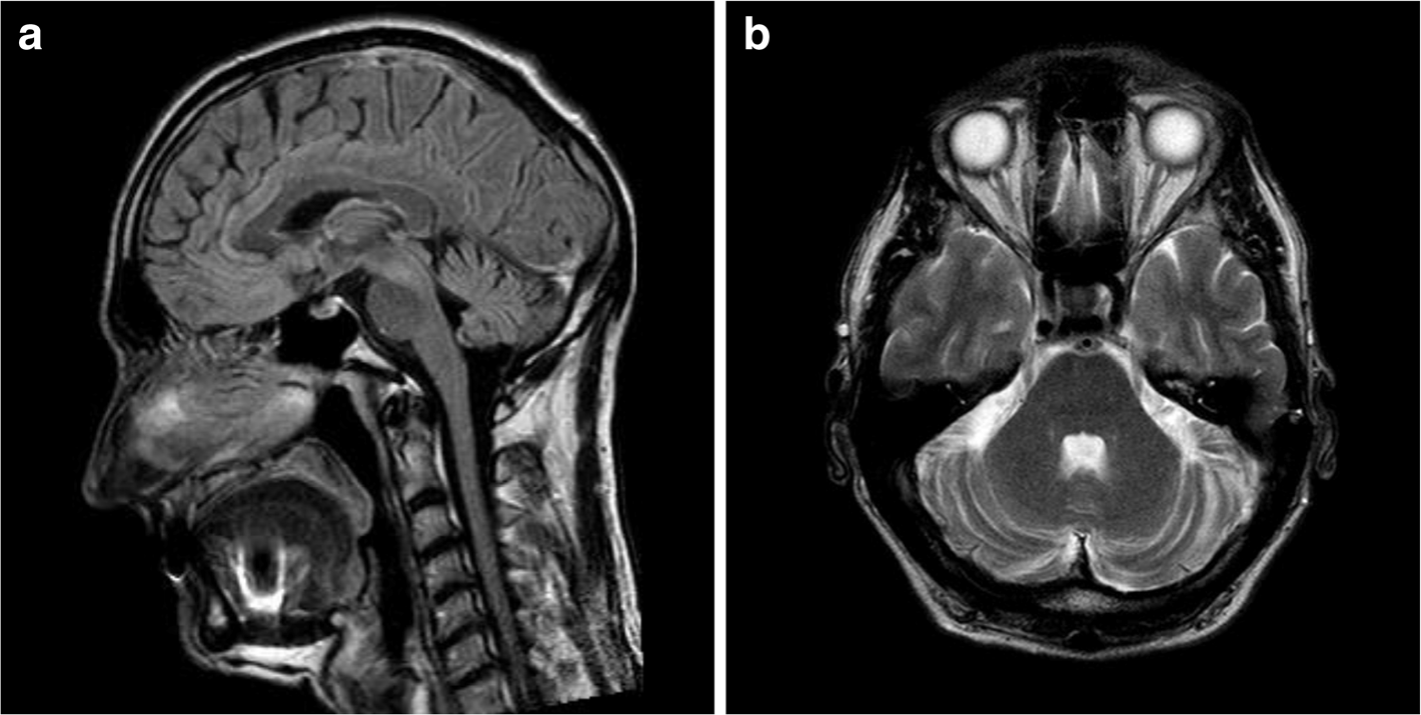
Cranial MRI of the proband (T2/FLAIR weighted sagittal a, and T2 weighted axial b images) showing mild cerebellar atrophy
